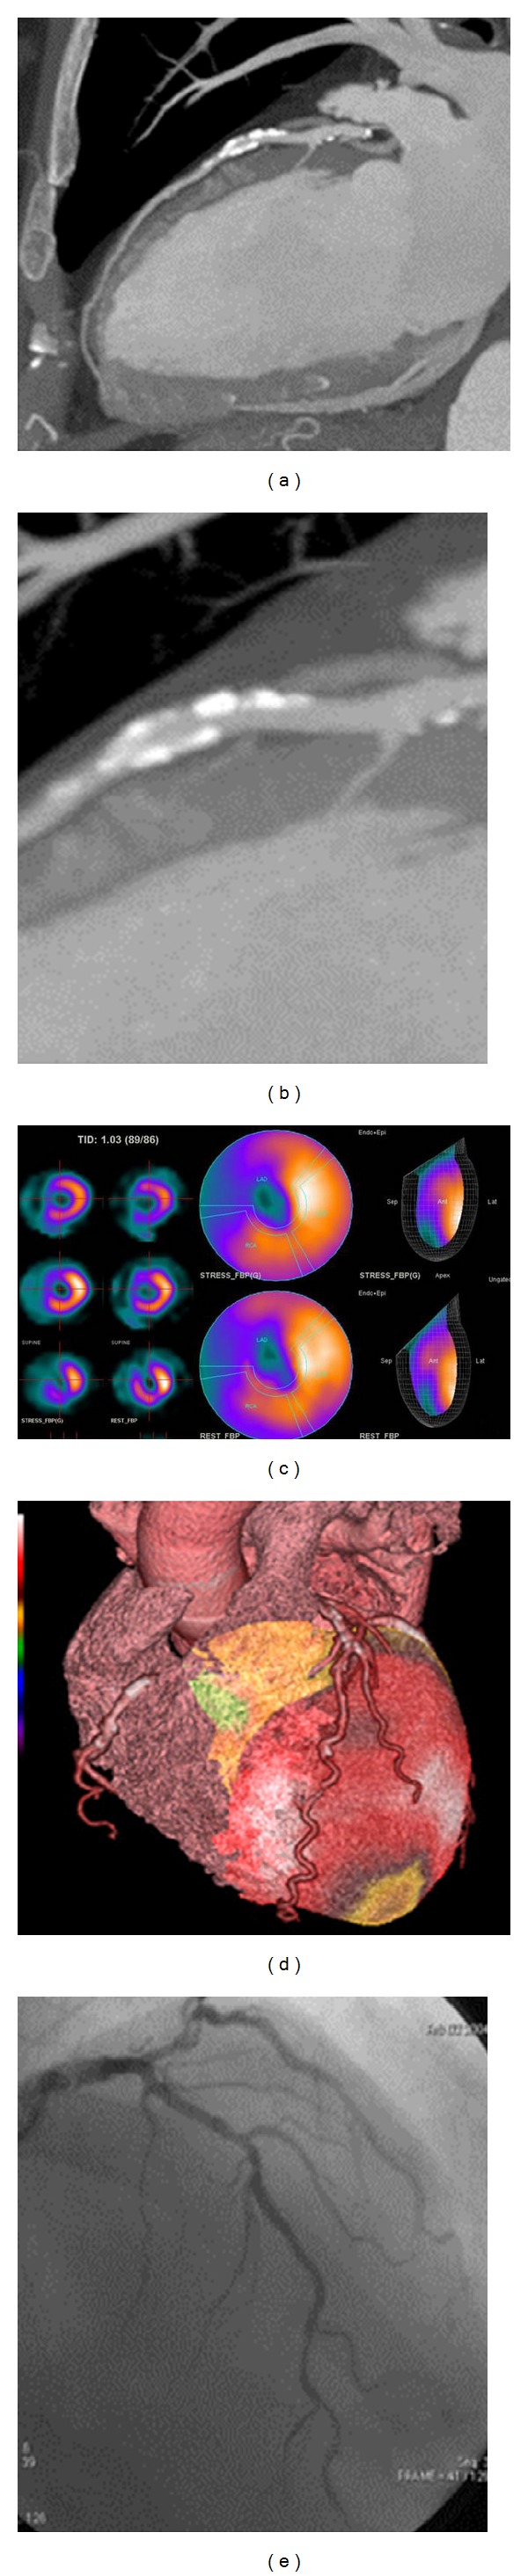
MPR reconstruction anterior descending artery and detail of multiple calcified in the proximal and middle parts ((a), (b)). SPECT images acquired after stress test and rest test showed a hypoperfusion of apex anterior-septal wall of left ventricle (c). Image fusion of the same case shows hypoperfusion of the area served by anterior descending artery (apex; anterior-septal wall) (d). Coronary angiography showed the presence of a stenosis at the middle third of the anterior descending artery (e).
